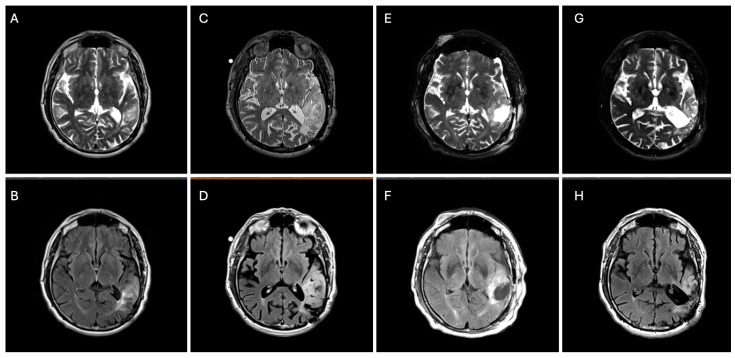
Axial T2 (top panels) and FLAIR (bottom panels) brain MRIs demonstrating an interval increase in left parietal–temporal T2/FLAIR-hyperintensity from 2017 (A,B) to 2024 (C,D) with subsequent surgical debulking (residual tumor volume 45 cm^3^) (E,F) followed by 8 months of vorasidenib (residual tumor volume 40 cm^3^) (G,H).
